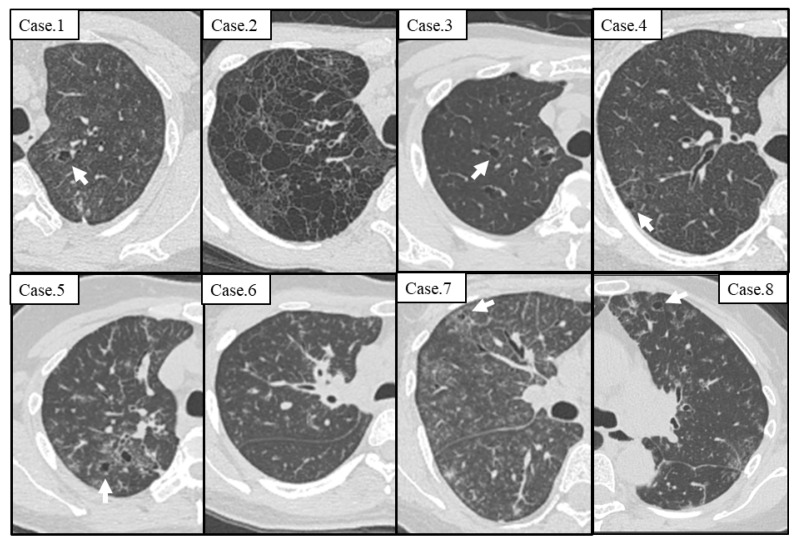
Cysts on Computed tomography at the first visit in 8 cases. Seven cases (87.5%) other than Case 6 had thin-walled cysts (arrow). There was ground-glass attenuation around cysts.
